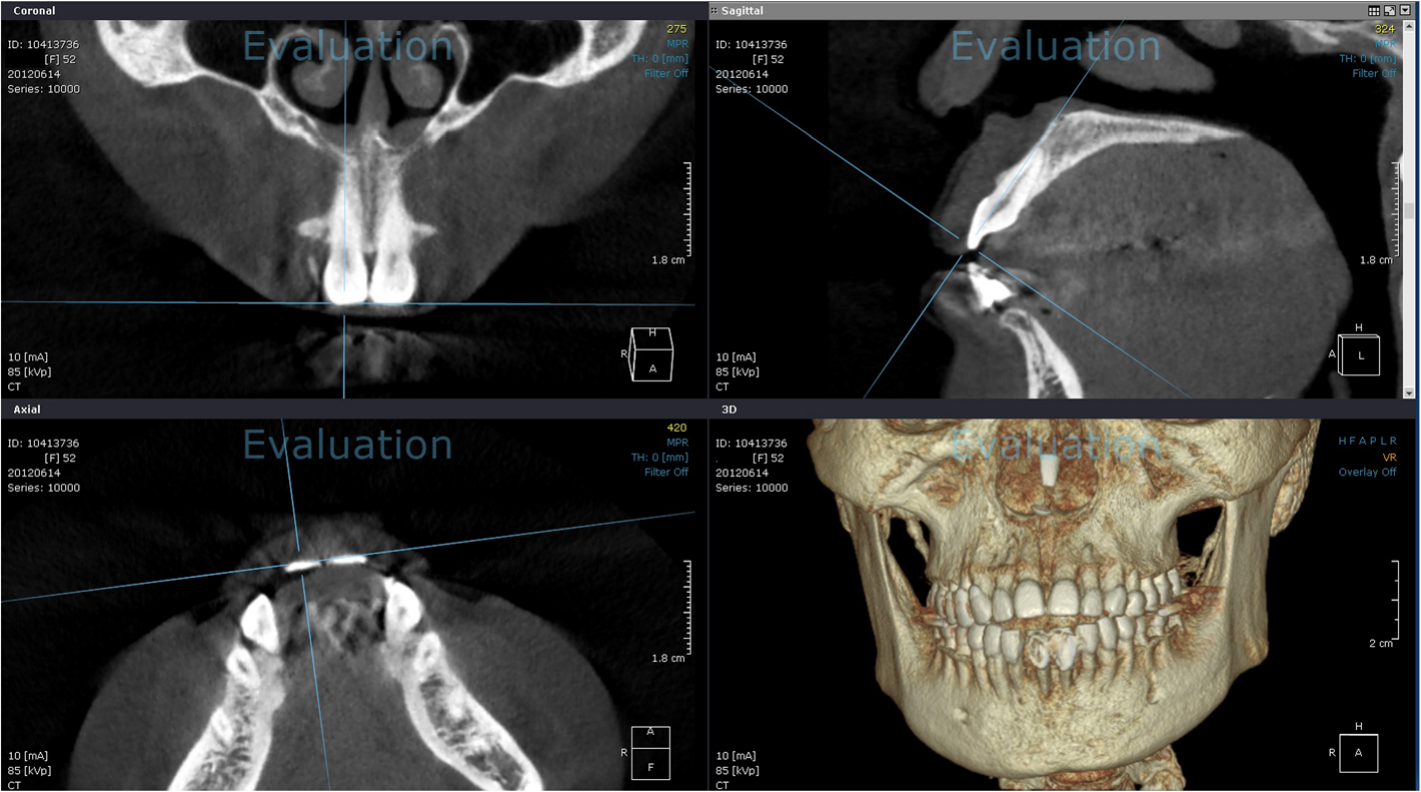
Tooth sectioning via cone beam computed tomography. The image was obtained by sectioning each tooth along the line connecting the midpoint of the incisal edge and the root apex, using software (OnDemand 3D)
